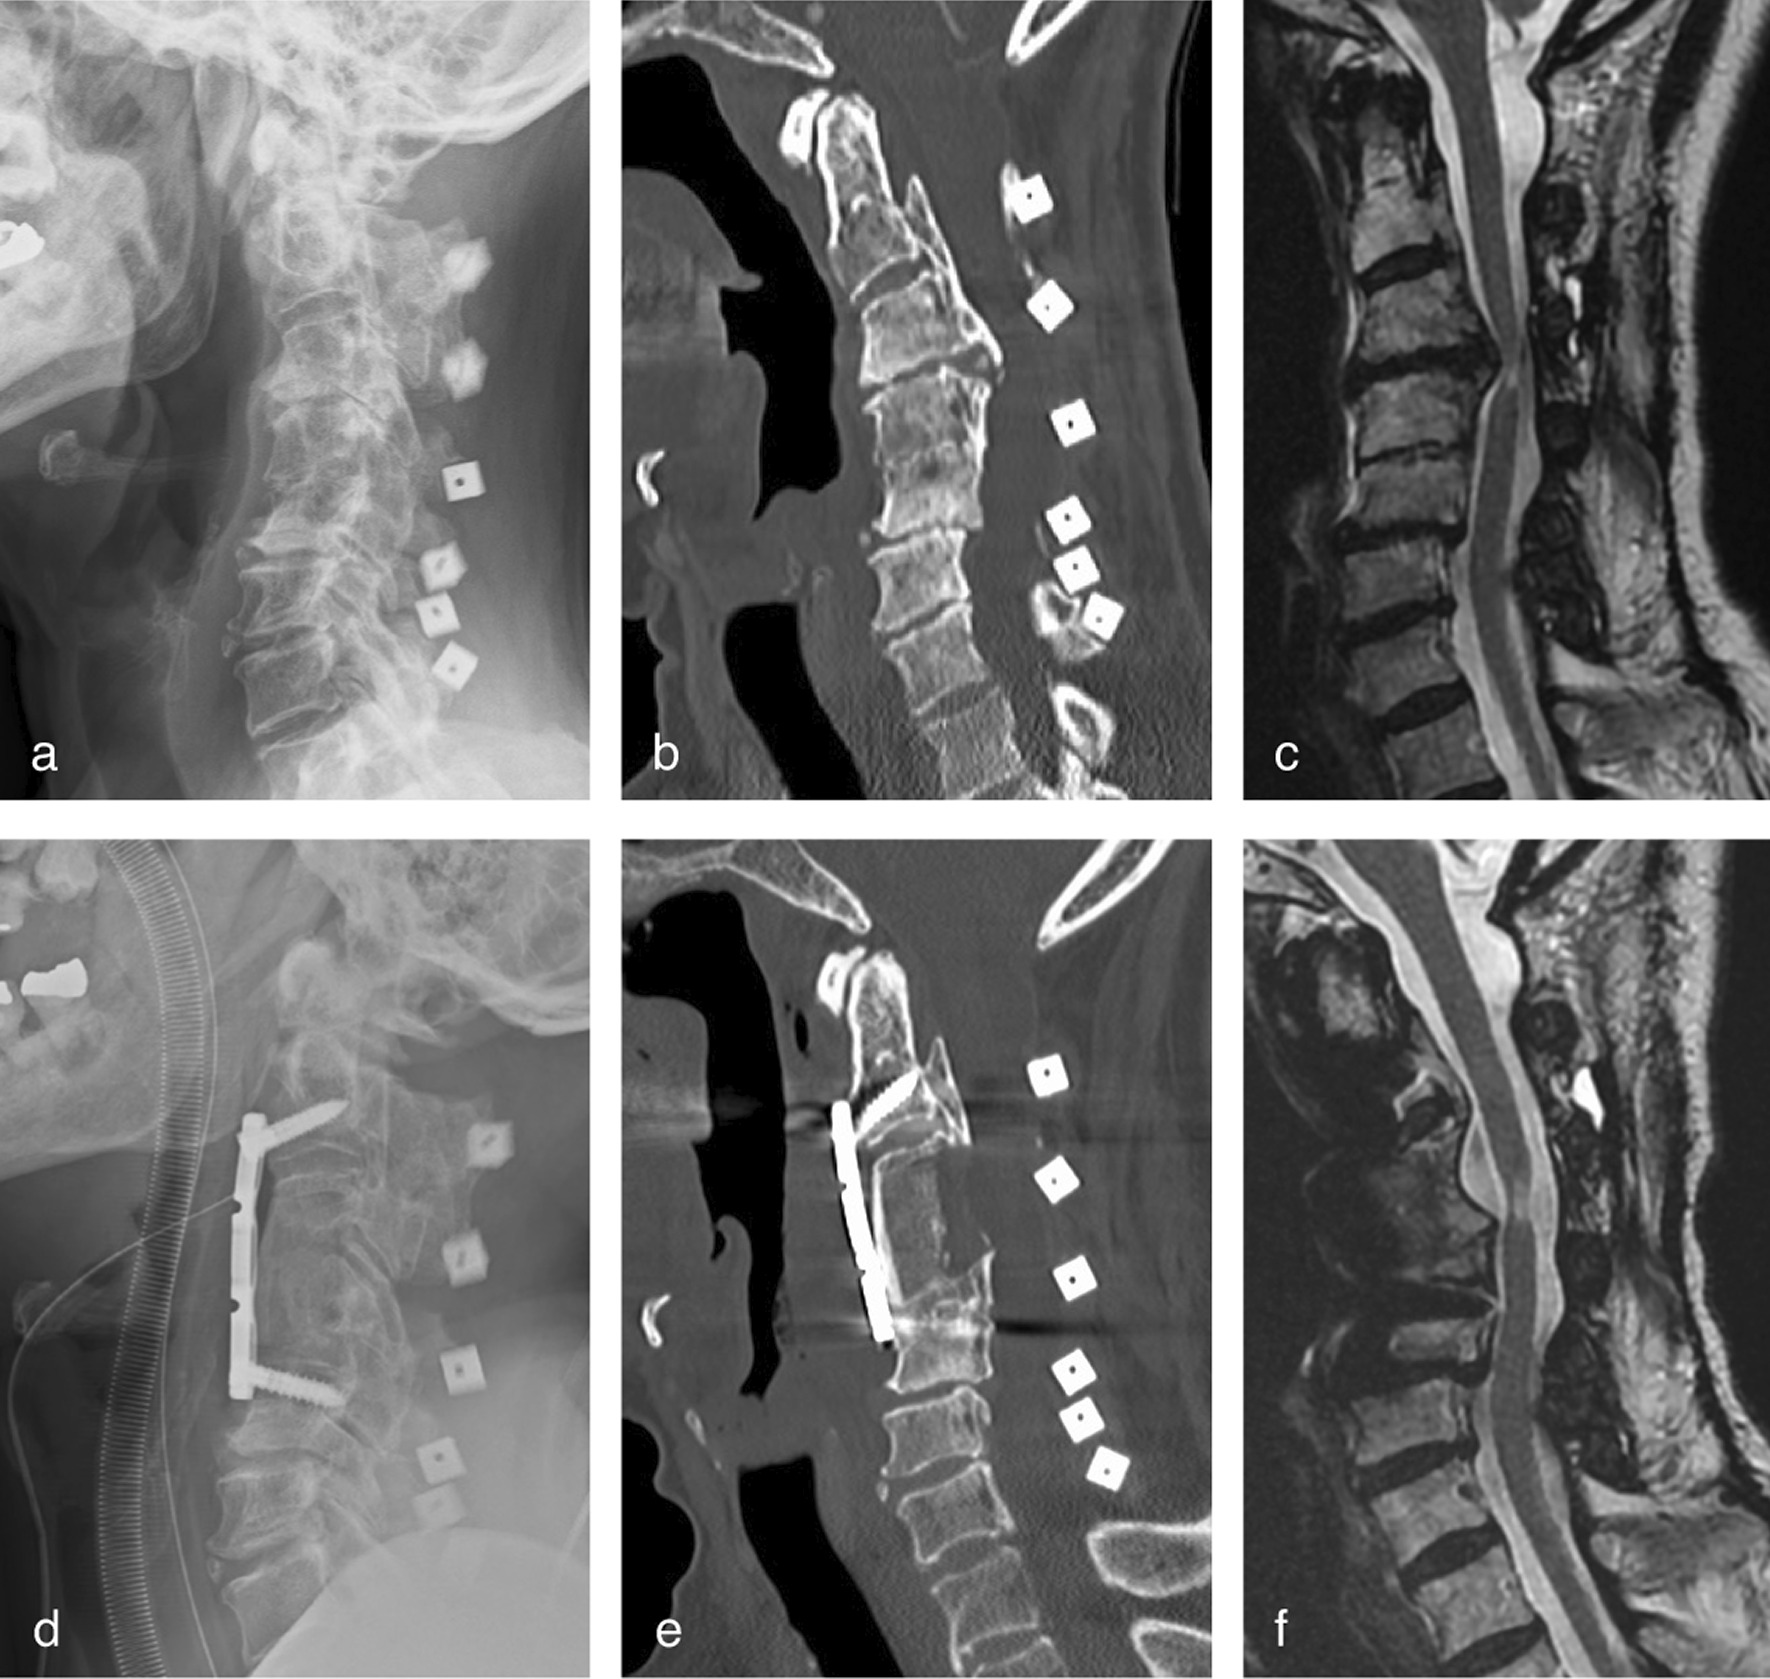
Case 11. a Preoperative lateral plain radiograph and b computed tomography sagittal image showing C2–5 continuous type ossification of the posterior longitudinal ligament (OPLL) and kyphotic deformity after laminoplasty. c Preoperative T2-weighed magnetic resonance sagittal image showing stenotic lesion with increased signal intensity of the spinal cord. d Postoperative lateral plain radiograph and e computed tomography sagittal image showing precise OPLL resection, iliac bone grafting, and C2–5 plate fixation. f Postoperative T2-weighed magnetic resonance sagittal image showing well-decompressed spinal cord
